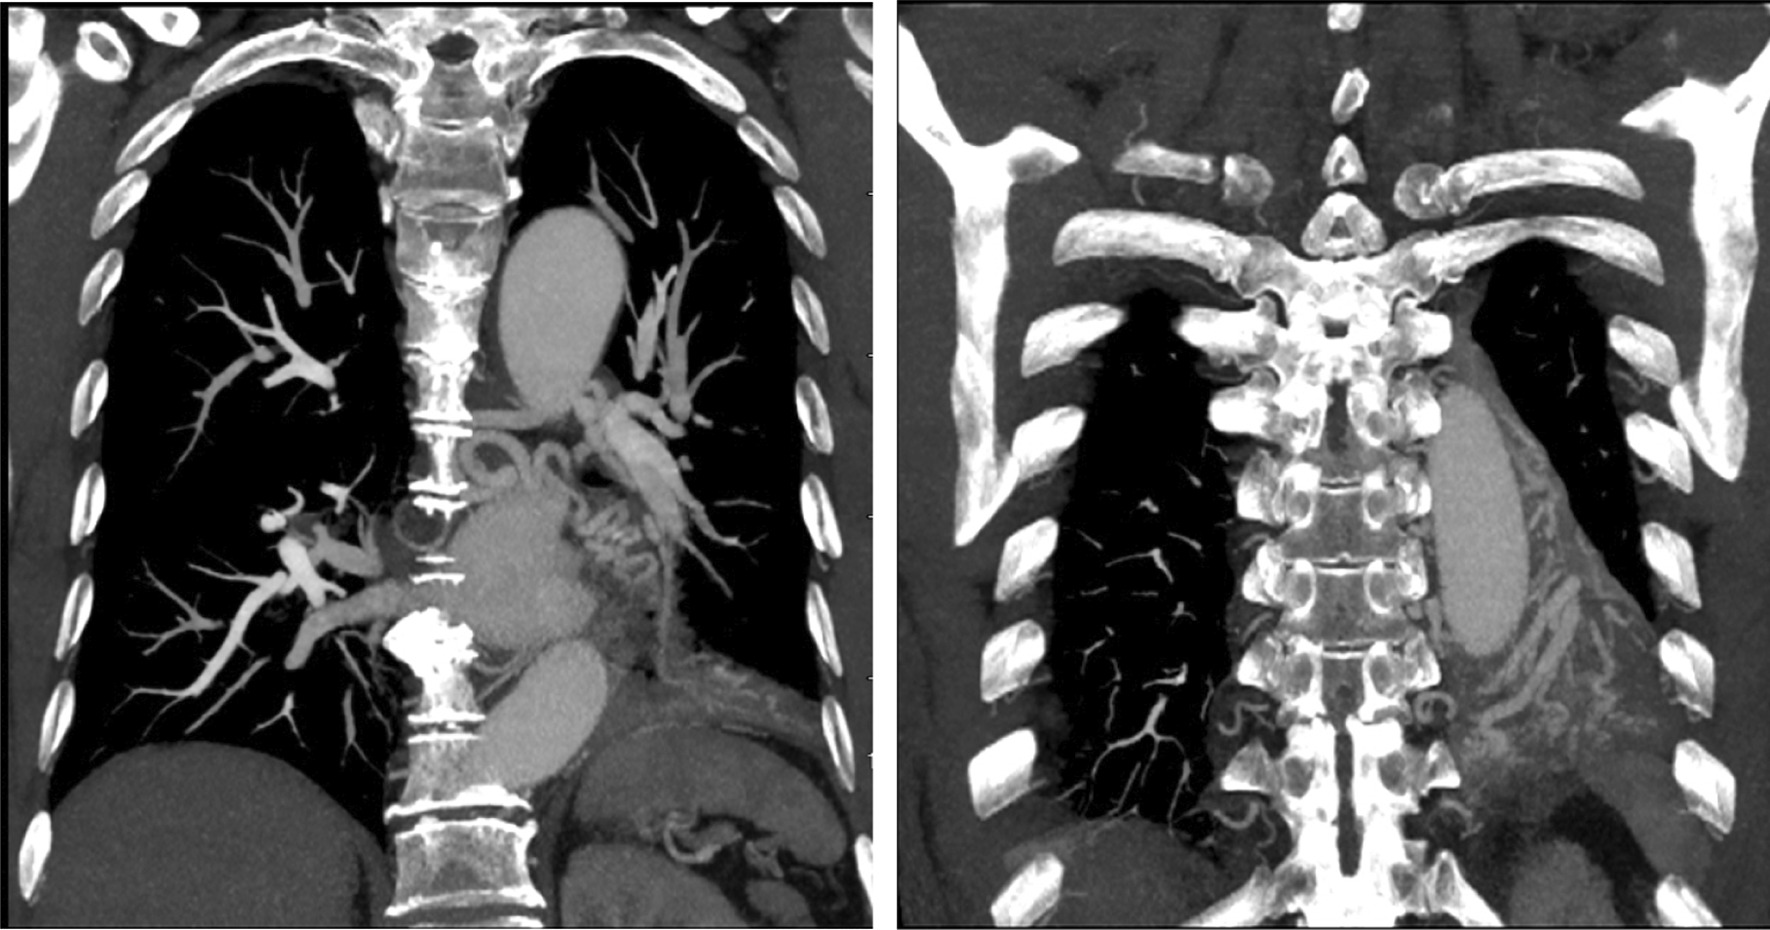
Computed tomography angiophy of bronchial artery (coronary plane): tortuous and dilated branches of left bronchial artery
